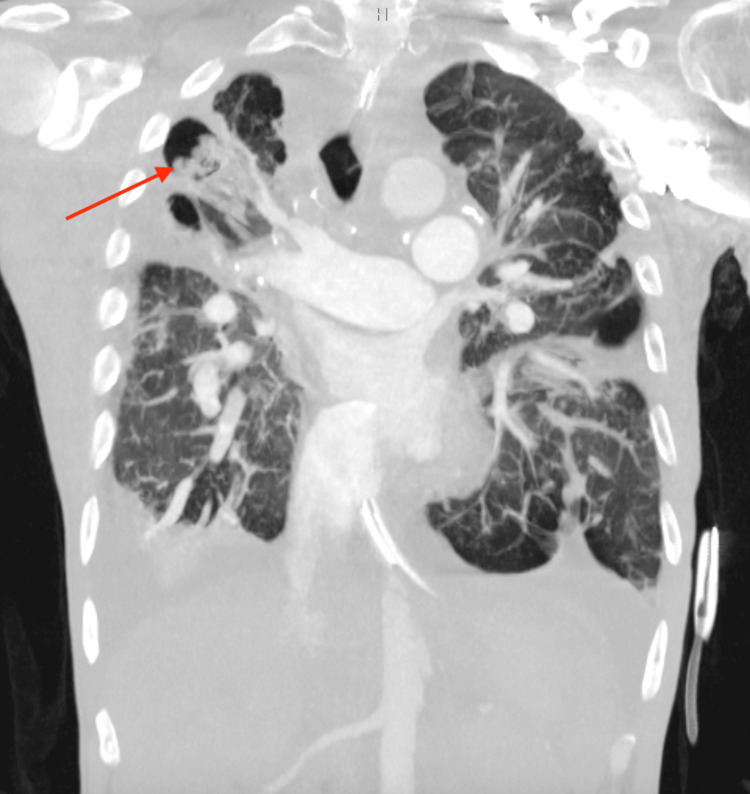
Computed tomography of the chest, anterior-posterior view, demonstrating fibrotic changes and a right upper lobe cavitation
